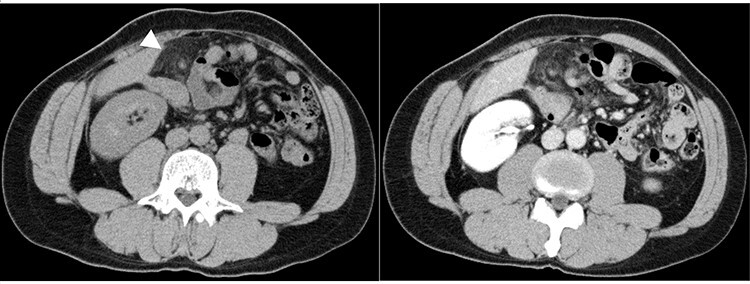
Abdominal CT images: nodular mass and high-density lesions in the fat tissue near the hepatic flexure of the ascending colon are evident (white arrow).
